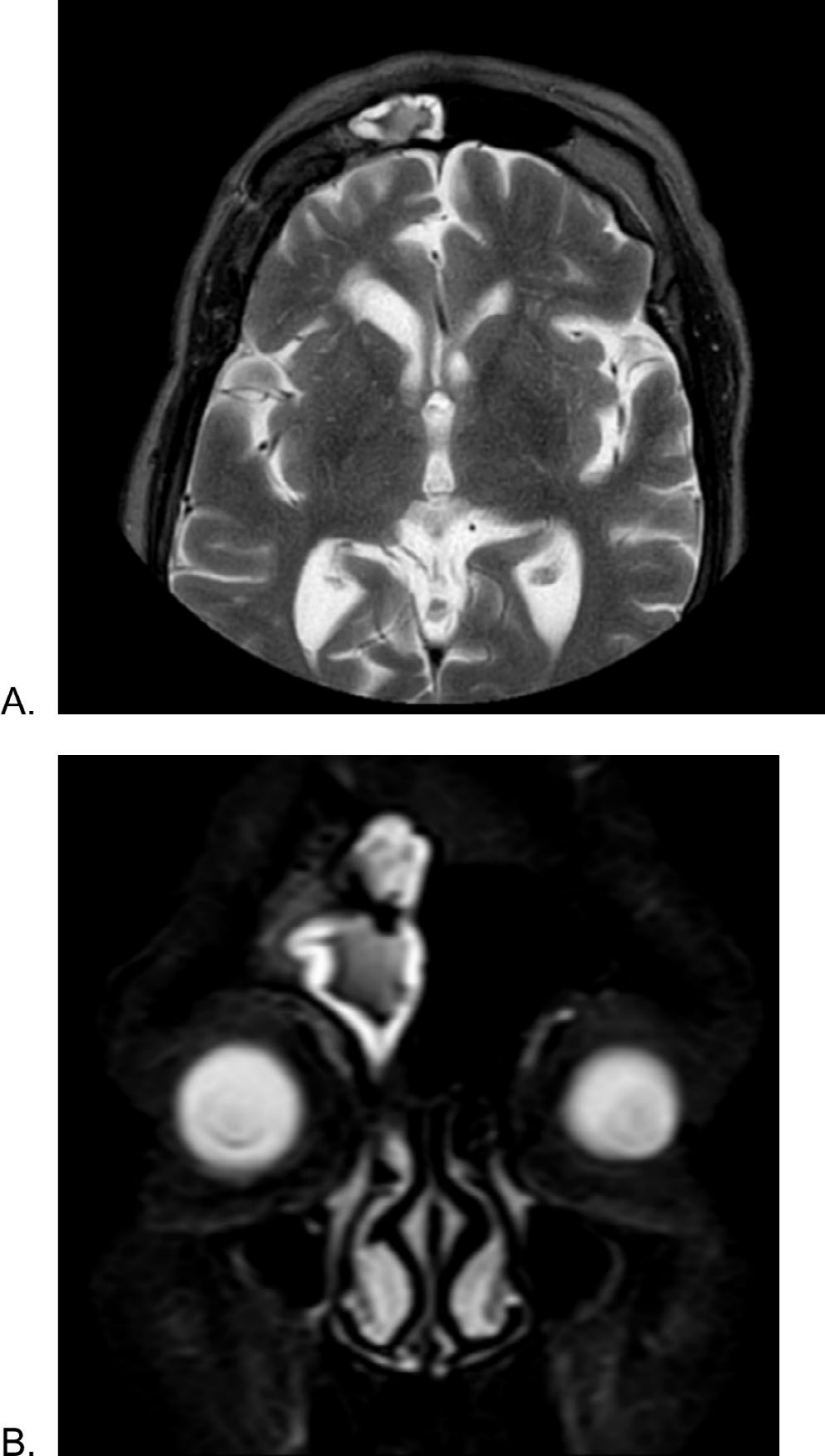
(A) Axial T2‐weighted MRI scan and (B) coronal T2‐weighted magnetic resonance imaging demonstrate opacification of the right frontal sinus, indicating fluid accumulation characteristic of sinusitis. Thinning of the anterior cortical wall is evident, which may signify osteomyelitis. Irregularities and erosions in the sinus walls could suggest a diagnosis of Pott's puffy tumor. Coronal T2‐weighted (B) reveals marked hyperintensity and expansion of the right frontal sinus. Evidence of mucosal thickening in the ethmoid sinus is also present. No parenchymal involvement is visualized. No evidence of intracranial complications is identified.
